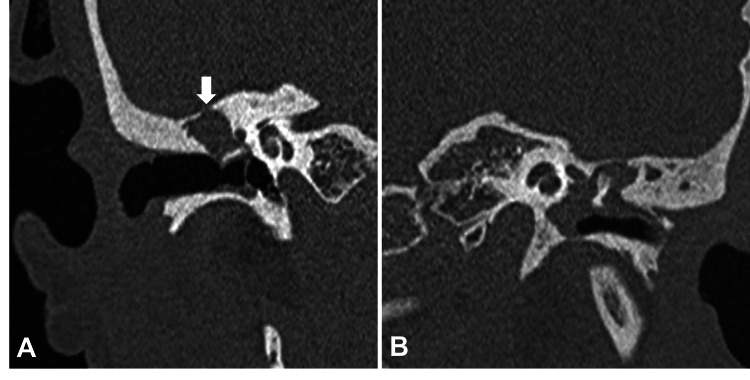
A reformatted coronal CT image of a patient which shows a non-dependent soft tissue density in the right middle ear (A) which is causing erosion of the tegmen tympani (white arrow). A coronal reformatted CT image of a patient which shows a soft tissue density in the left middle ear (B) which is causing erosion and deossification of the head of malleus.
